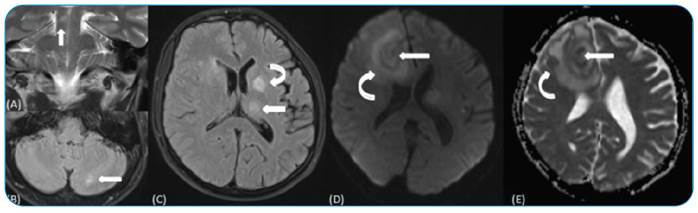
(A) Coronal T2-weighted image demonstrates an indistinct intense lesion on the cerebral peduncle (arrow); (B) axial FLAIR image shows similar focus on the left cerebellar hemisphere (arrow); (C) axial FLAIR image indicates thalamic (arrow) and putaminal (curved arrow) intense lesions; (D-E) and DWI and apparent diffusion coefficient map reveals restricted diffusion within the lesion and capsule (arrow), and extensive vasogenic edema (curved arrow). DWI: diffusion weighted imaging; FLAIR: fluid-attenuated inversion recovery.
